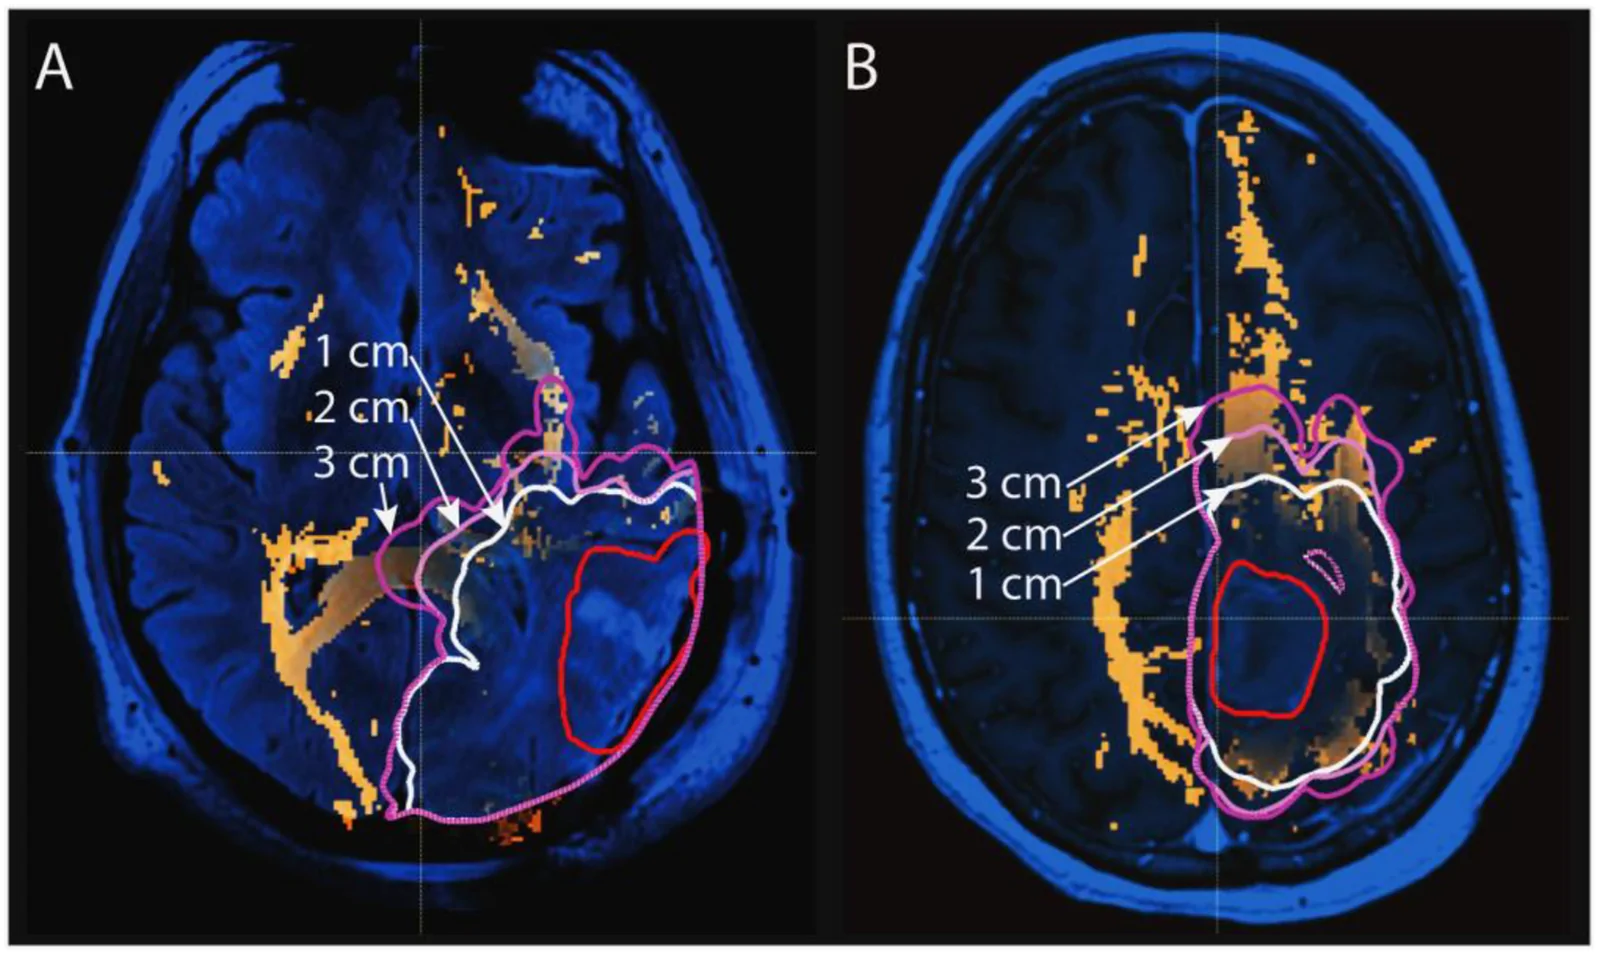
Anisotropic CTV expansions for two patients (A: patient 3, an occipital-temporal lesion, B: patient 7, a mostly parietal lesion, from Table 1) using white matter path length maps displayed in orange over post-contrast T1 weighted MRI in blue
